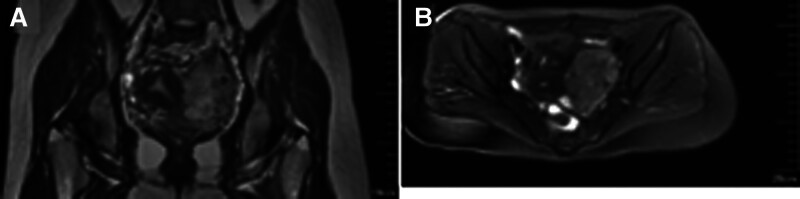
Pelvic MRI: (A) T2-weighted image showing a lobulated appearance of the pelvic occupancy; (B) MRI image of the pelvic occupancy with fat suppression technique applied. MRI = magnetic resonance imaging.
